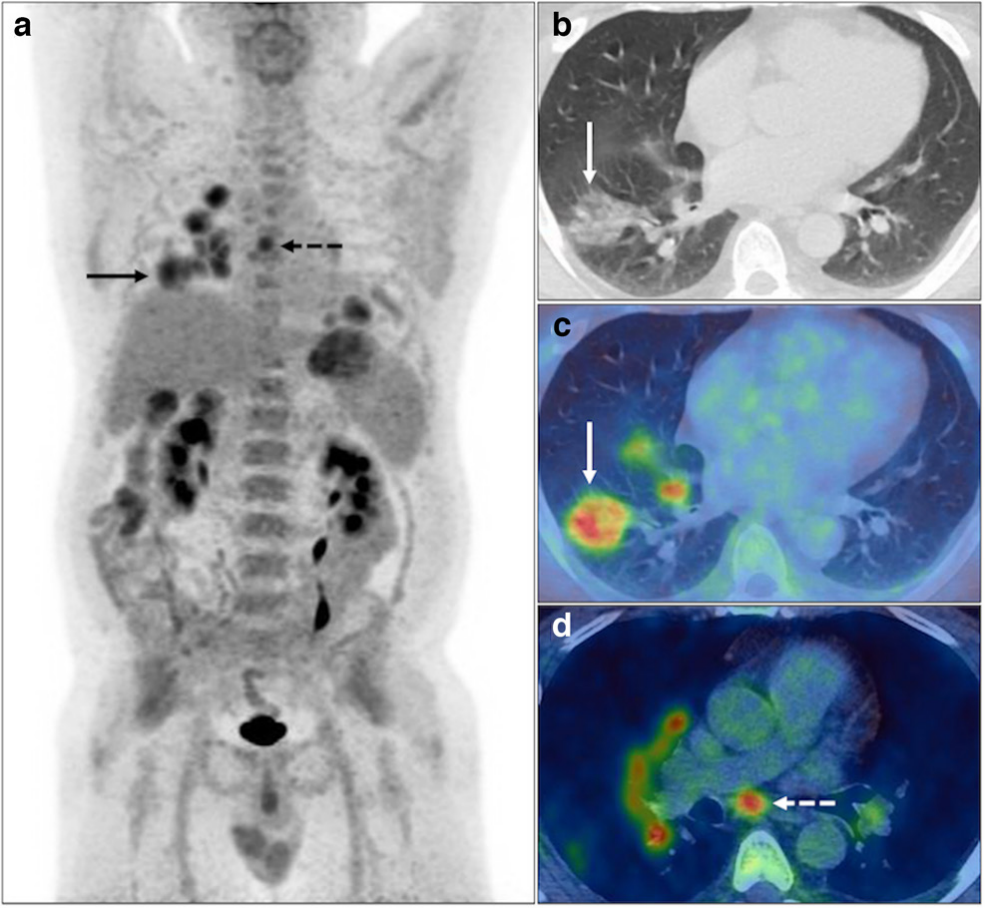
Bilateral (right>left) FDG avid (SUV_max_ 8.7) nodular consolidation including foci of central GGO with surrounding circumferential consolidation, *i.e*. ‘reverse-halo’ sign, in the right lower lobe (solid black and white arrows), with reactive non-enlarged FDG avid (SUV_max_ 6.5) subcarinal lymph node (dashed black and white arrows) on PET MIP (A), axial CT and fused PET-CT (B-D). BSTI 1 with positive RT-PCR (*confirmed* case).
